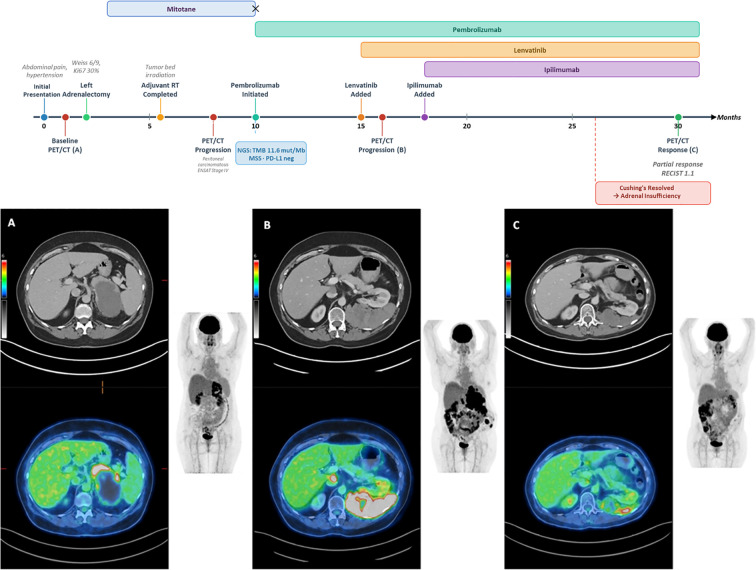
Clinical timeline and 18F-FDG PET/CT scans of ACC; representative maximum intensity projection (MIP), axial CT, and fused PET/CT images demonstrating disease staging (A) progression (B) and therapeutic response (C). (upper panel) A clinical timeline summarizing the key diagnostic, surgical, and therapeutic milestones from initial presentation through the current follow-up. (A) baseline imaging reveals a large, heterogeneous left adrenal mass characterized by a central necrotic core and intense peripheral pathological uptake, consistent with primary adrenocortical carcinoma. (B) follow-up imaging demonstrates significant disease progression. Extensive FDG-avid lesions are noted within the adrenal bed, accompanied by widespread metastatic involvement including abdominal and pelvic lymphadenopathy, as well as peritoneal spread. (C) Post-treatment evaluation shows a favorable partial response per RECIST 1.1, with significant metabolic improvement. There is a notable reduction in tracer avidity and a partial regression of the local recurrence and the majority of previously identified metastatic lesions.
